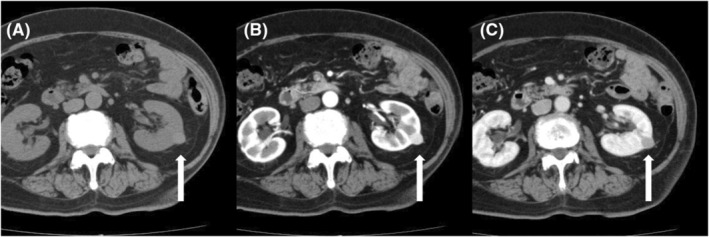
Abdominal computed tomography (CT) images showing the left renal tumor. (A) Unenhanced CT scan shows a homogenous and hyperdense area compared with the normal renal parenchyma. (B) Enhanced CT scan shows homogenous enhancement in the early phase. (C) Enhanced CT scan shows washout in the late phase. White arrows indicate the left renal tumor.
